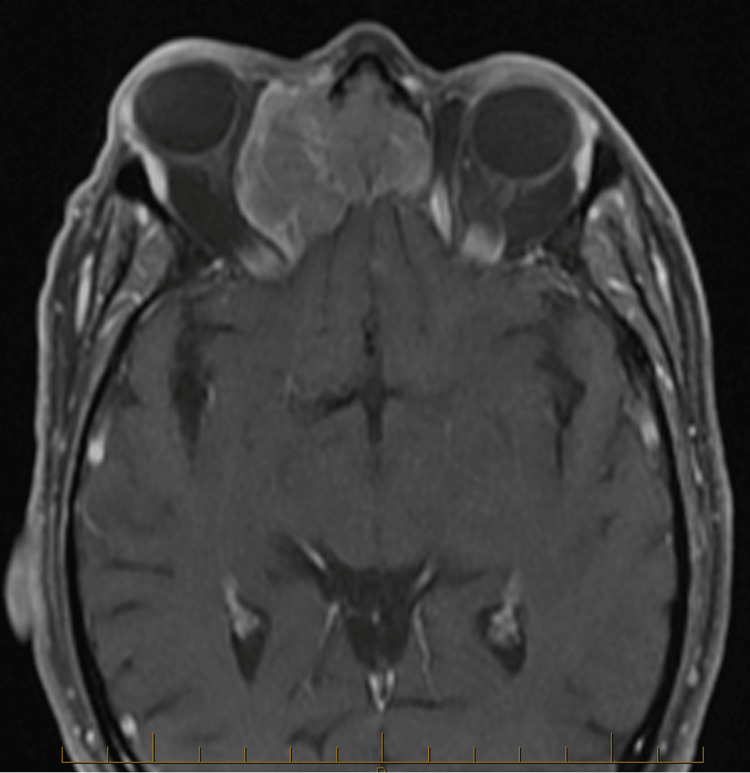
MRI orbit T1+c acquired on April 25, 2024. MRI: magnetic resonance imaging
